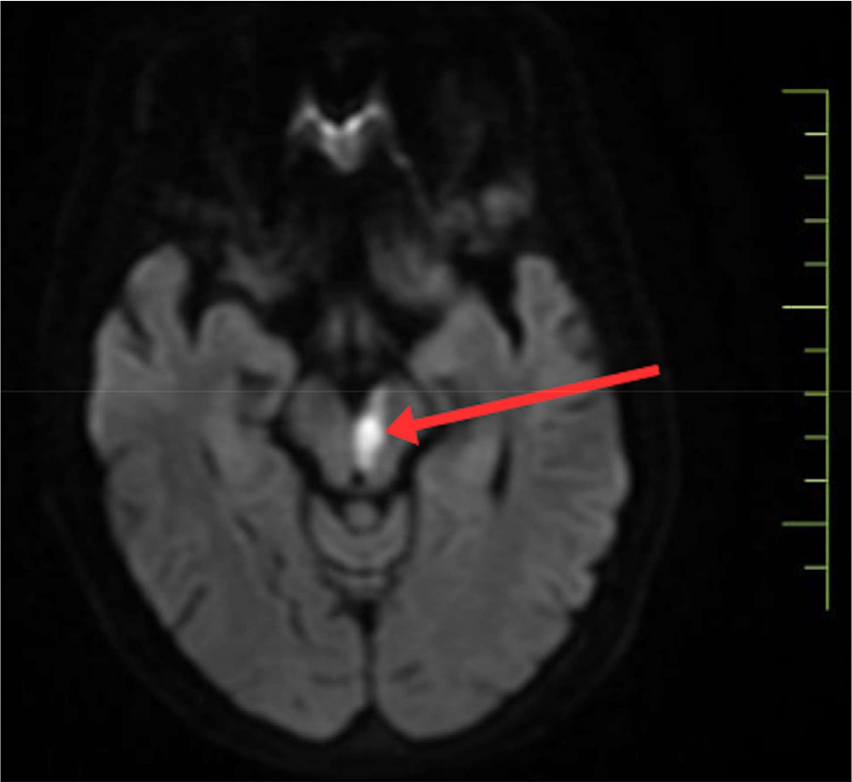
Shows the left paramedian midbrain's hyperintense area on diffusion-weighted imaging on MRI axial sections, indicating an acute infarct.
